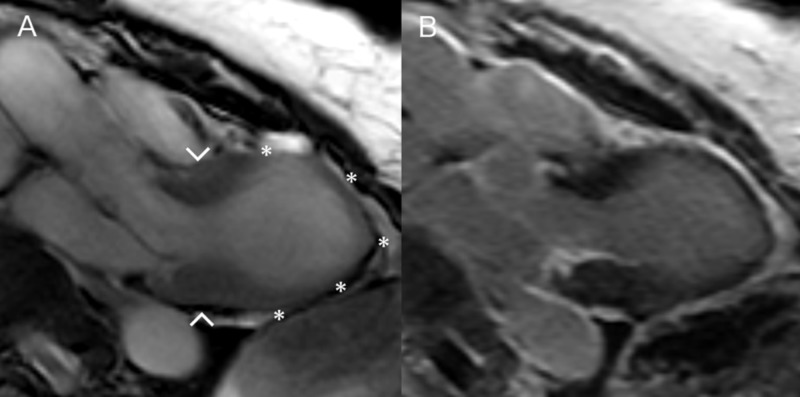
Cardiac MRI of our patient A: Systolic image with akinesia of the mid-ventricular and apical segments of the left ventricle (asterisks) with preserved contraction in the basal segments (arrowheads). B: Absence of late gadolinium enhancement.
